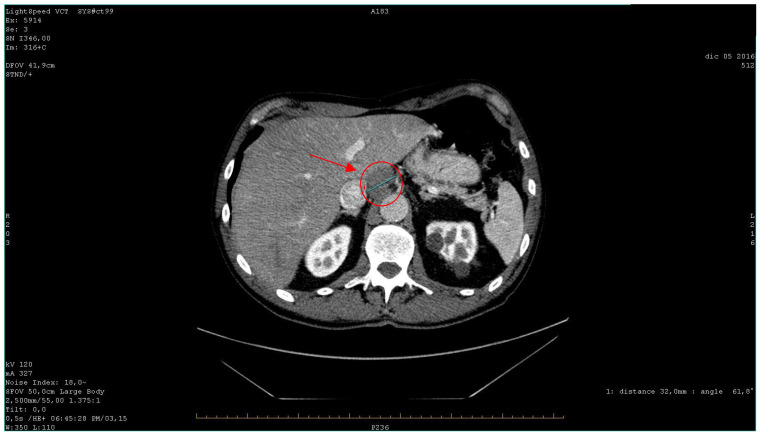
The axial CT scan of abdomen with contrast medium shows tripod lymphadenopathy (marked with a red circle) (2016).
